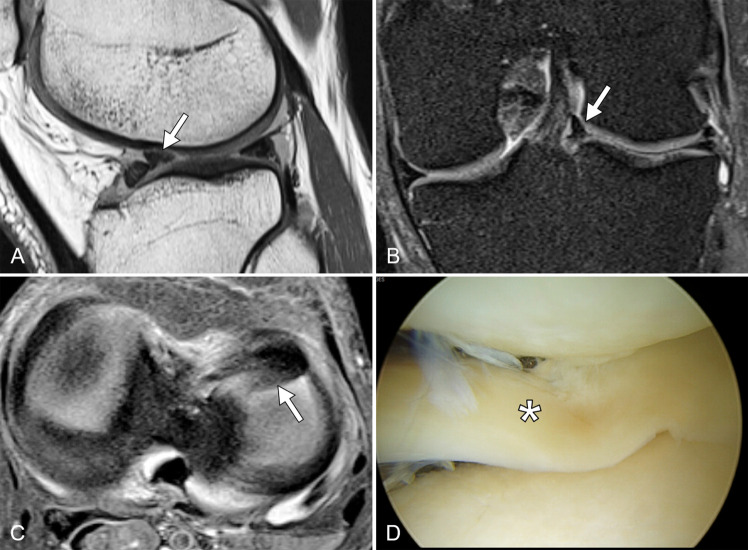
Bucket-handle tear of the lateral meniscus in a 36-year-old man. Sagittal proton-density-weighted MRI (A), coronal proton-density-weighted fat-suppressed MRI (B), and axial proton-density-weighted fat-suppressed MRI (C) show central fragment (arrows) displaced into the intercondylar notch. Arthroscopic view (D) demonstrates large lateral meniscus fragment (*) displaced anteriorly and medially located in the intercondylar notch
